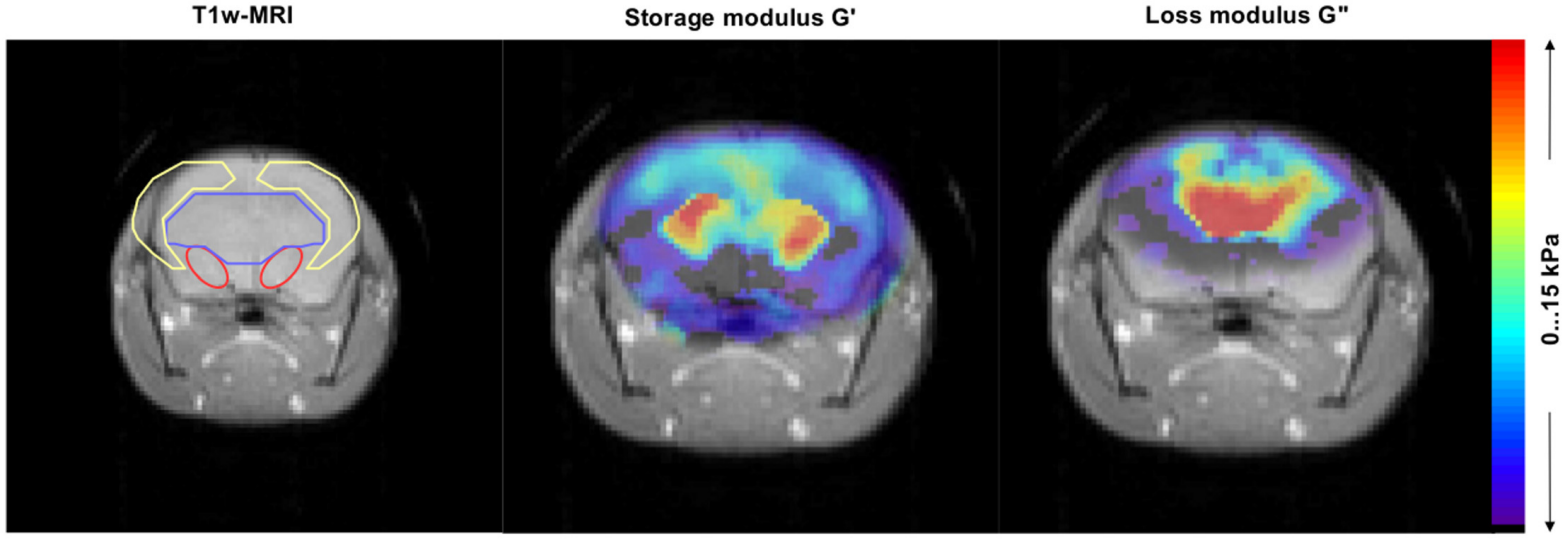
Representative images of MRI signal and complex modulus map of G' and G''. Regions of interest: substantia nigra (red line), midbrain (blue line) and hippocampus (yellow line) were marked in T1w-MRI.
